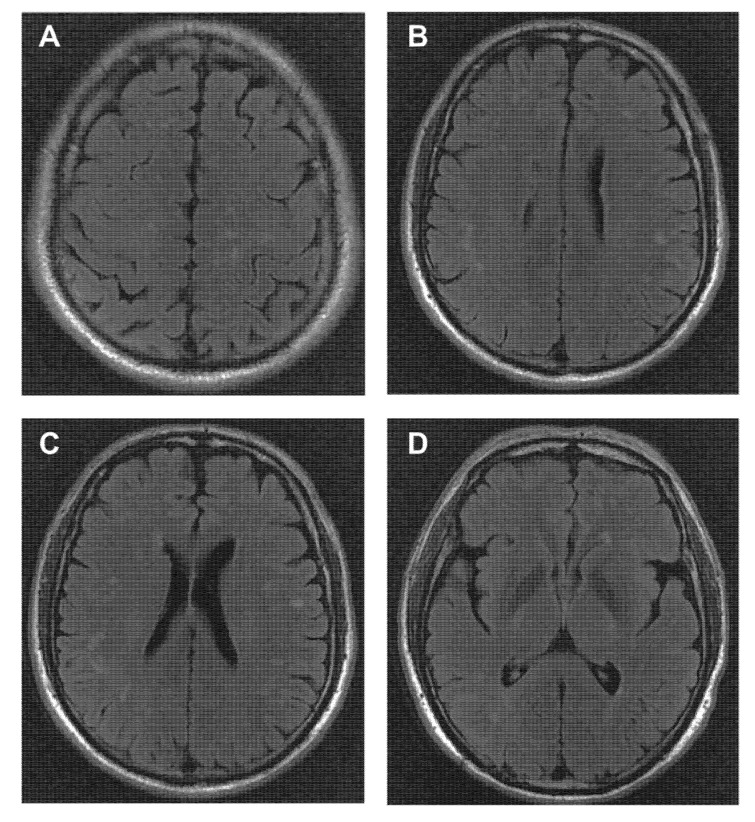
Radiologic features of MOG-IgG-positive patients with cranial neuropathies. T2-FLAIR hyperintensities in the subcortical white matter of bilateral frontal lobe (A); bilateral frontal and parietal lobe (B,C); and right occipital lobe near the posterior horn of the lateral ventricle (D).
